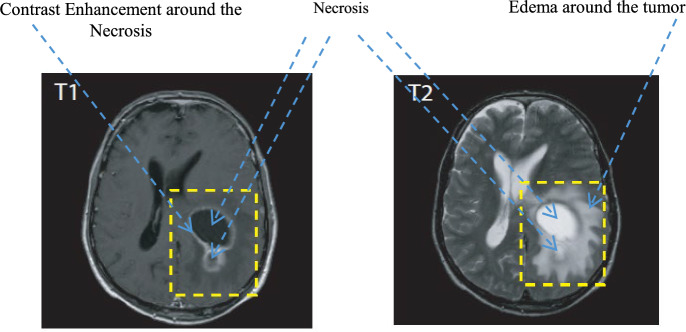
The MRI images of Grade IV glioma exhibit distinct characteristics in terms of the morphology of the brain tumor. These characteristics include the presence of tumor enhancement in the T1 images after contrast enhancement. The center of the tumor is marked by necrosis, while edema surrounds the tumor and is visible in the T2 images.
